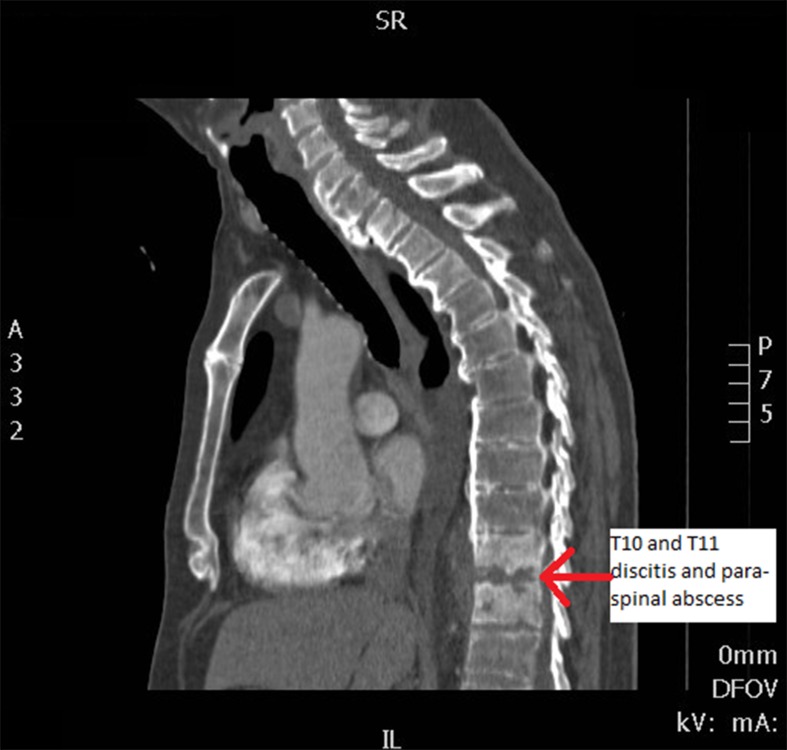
Sagittal computed tomography of the thorax showing T10 and T11 discitis with paraspinal abscess
